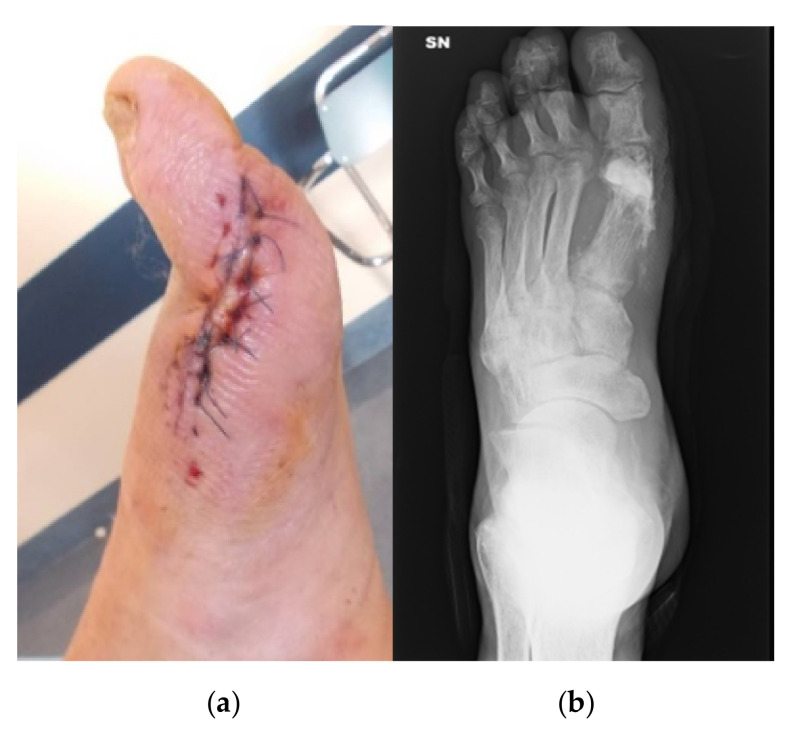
Post-operative photograph (a) and X-ray (b).
